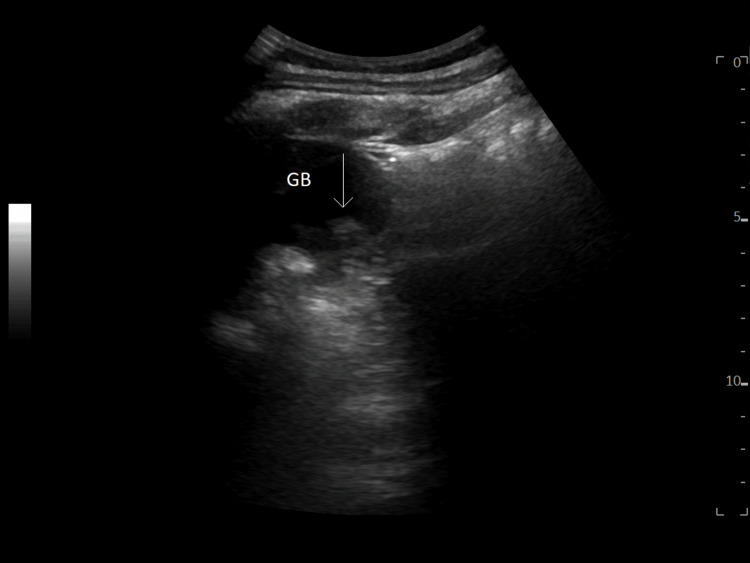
Ultrasound of the liver revealing hypoechoic structure in the gallbladder (later determined to be an artifact) without any other abnormalities. The arrow indicates the hypoechoic structure later determined to be an artifact. GB: gallbladder.
